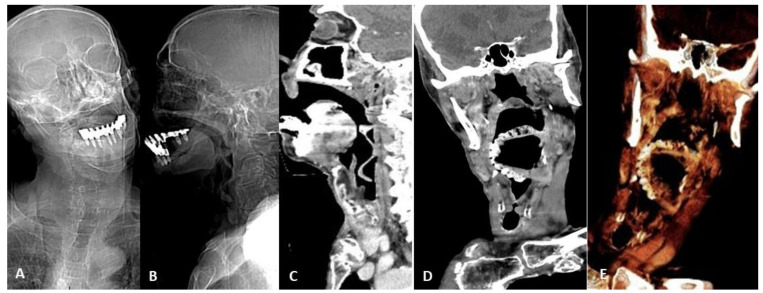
Device migration. Eighty-seven-year-old man affected by Alzheimer disease and asphyxiation crisis. Frontal (A) and lateral (B) head and neck CT scout views show denture migration with hypopharynx impaction. Contrast-enhanced CT sagittal (C) and coronal (D) scans better show denture relationship with the surrounding structures and demonstrate anterior epiglottis displacement with subsequent airways restriction (arrow). 3D VR image thin slab (5 mm) view (E) better shows device migration.
